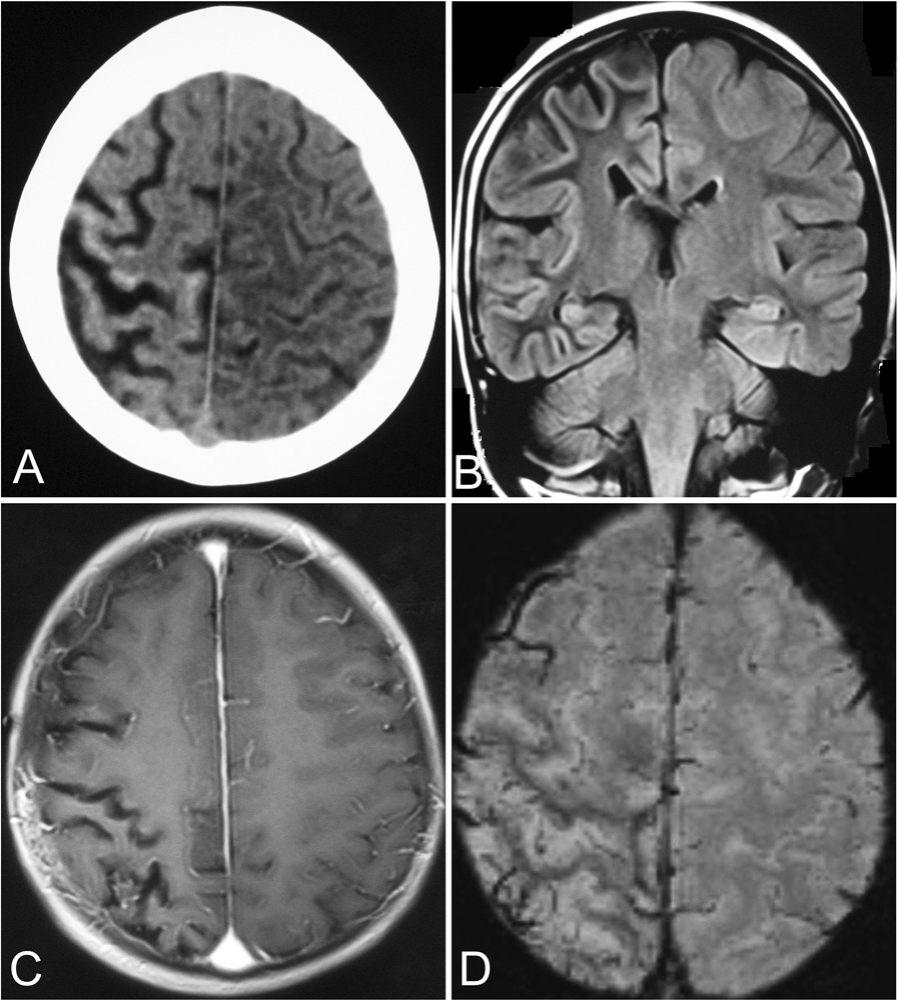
Radiological characteristics of brain. CT shows cortex atrophy and gyral calcifications in right parietal lobe (A); coronal MRI shows the right side of the cerebral cortex atrophy (B); enhanced MRI reveals mild leptomeningeal angiomatosis in right temporal-parietal areas (C); SWI shows vein hyperplasia in the atrophic cerebral sulcus (D).
